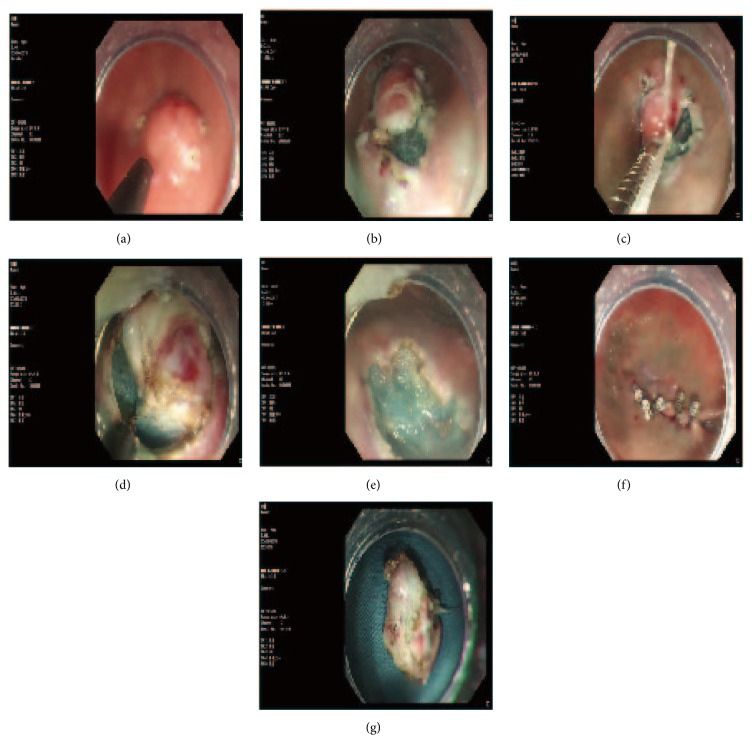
ESD with dental floss clip traction. (a) A bump was seen in the gastric fundus (endoscopic ultrasound showed it originated from the muscular layer, 1.5cm in diameter), labeled with Hook Knife. (b) Hook knife cut off most part of the mass along the labeled margin, while the endoscopic transparent cap could not enter into the gap between the lesion and normal tissue, which led to difficulty of the resection. (c) The clip fixed the dental floss right in front of the endoscopic vision. (d) After the traction, the lump was clearly defined by the normal tissue, and the HK knife was easy to peel off the lump. (e) The post-ESD wound has no defect left. (f) The wound was closed with titanium clips. (g) The mass.
